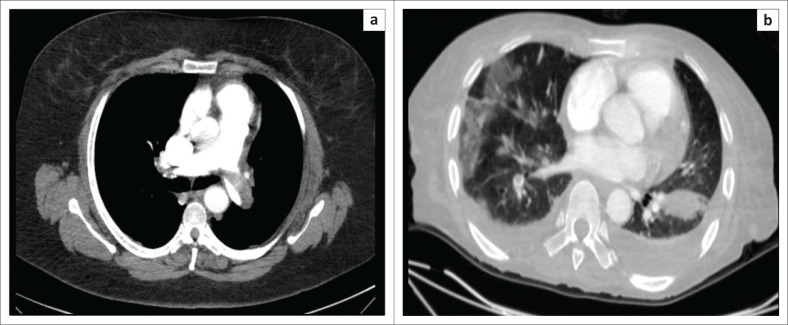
Axial CT of two different patients with pulmonary embolism. (a) Demonstrating a large saddle embolus; (b) demonstrating a filling defect in the right descending pulmonary artery complicated by bilateral pleural effusions, peripheral consolidations and a wedge-shaped pulmonary infarct on the left.
